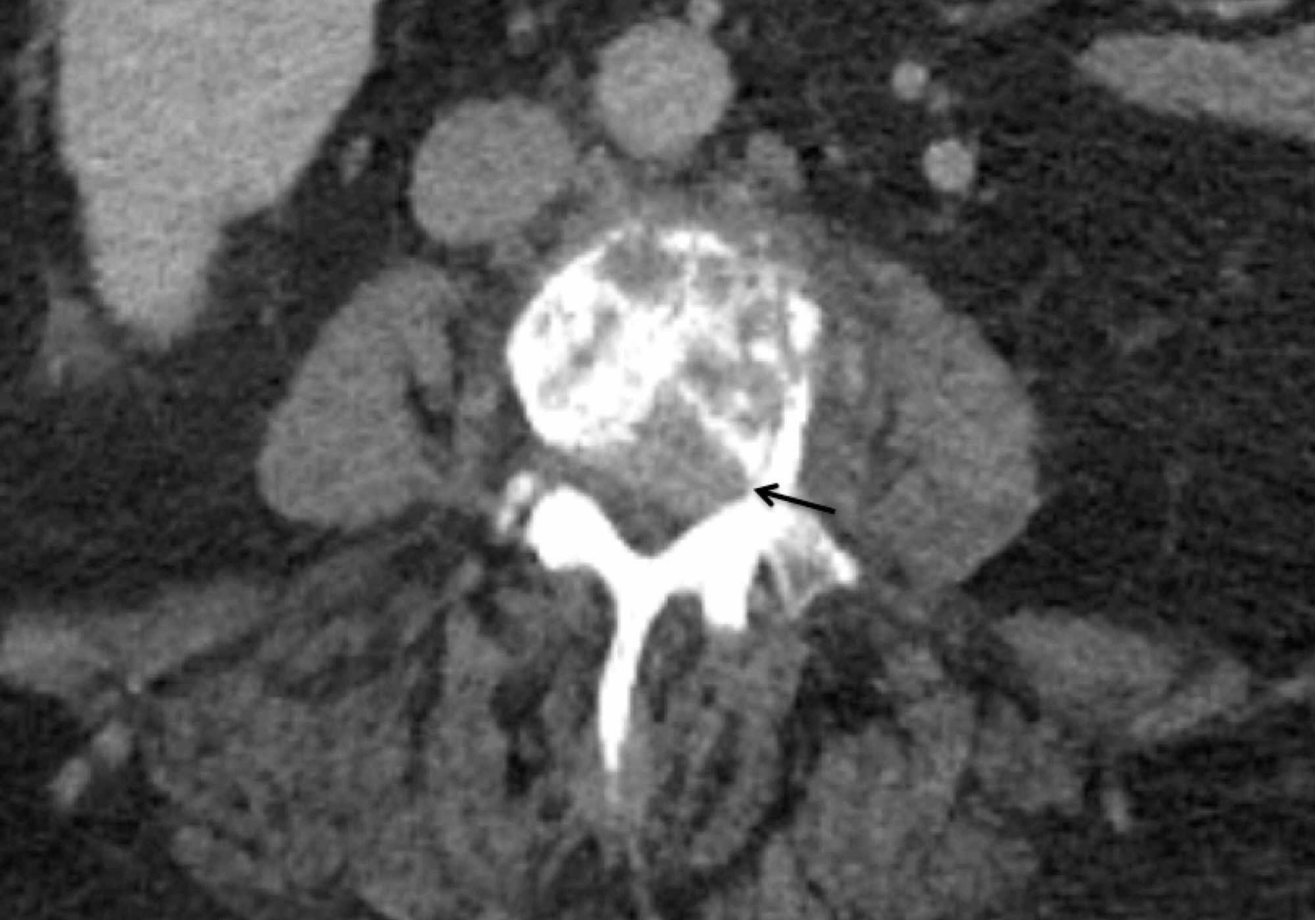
Pre-treatment portal venous phase CT demonstrating a destructive lesion within the L4 vertebral body with enhancing epidural soft tissue tumour extension (black arrow) resulting in moderate central canal stenosis.
